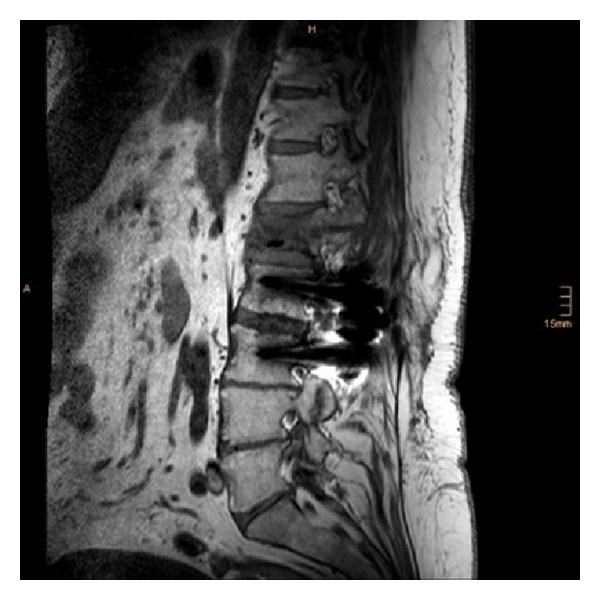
Postoperative sagittal MRI (T1-weighted) at the level of the pedicles (left).
